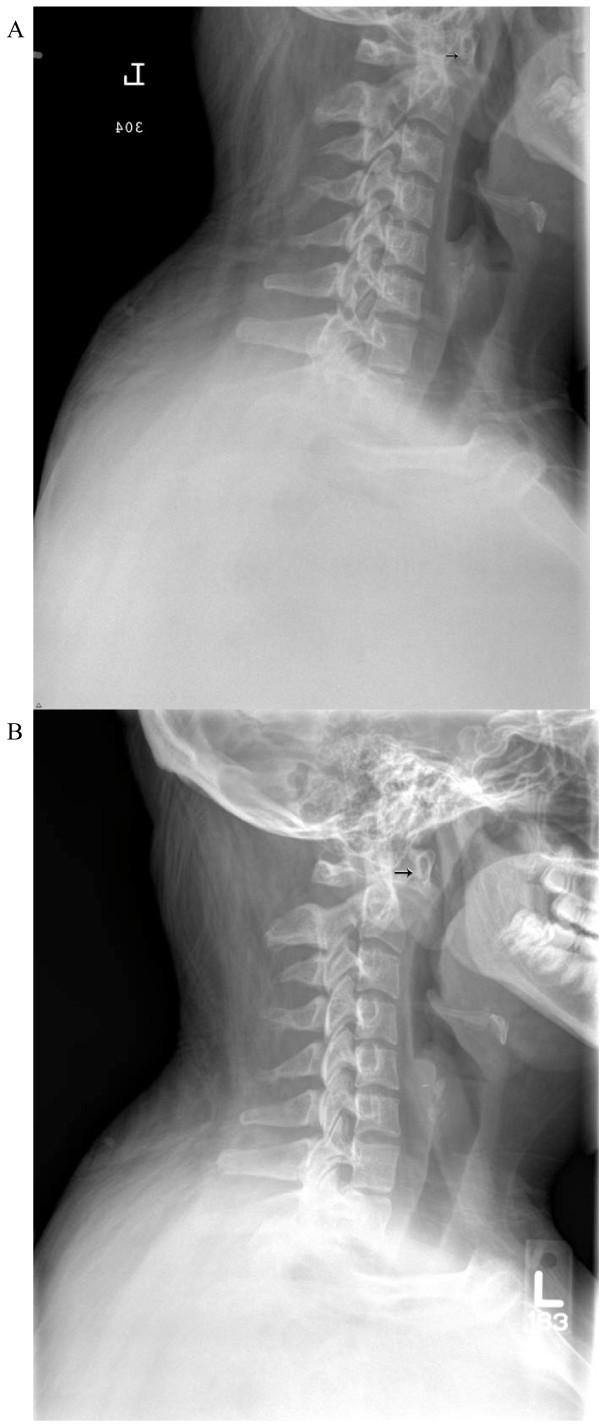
A) Lateral cervical spine X-ray showing an atlantodental interval (arrow) of less than 6 mm. B) Lateral cervical spine X-ray shows a progressive widening of the atlantodental interval (arrow) to more than 10 mm in the span of three months.
